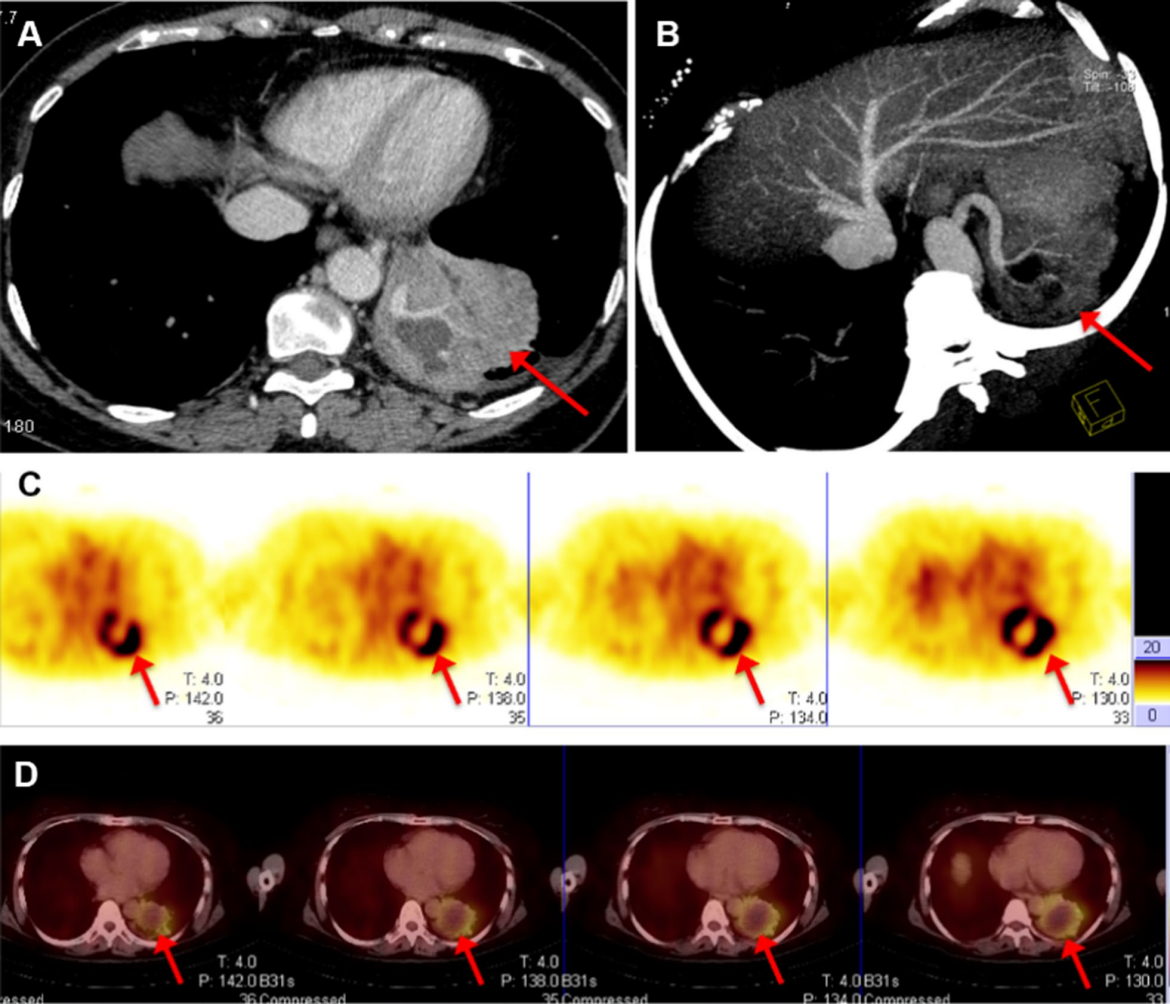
[^99m^Tc]Tc-Galacto-RGD_2_ imaging showed focal uptake in a patient with pulmonary sequestration. a, b Enhanced CT showed the tumour blood supply originated from the abdominal aorta. c, d RGD imaging showed focal uptake in the tissue, and necrosis in the central zone of the tissue
